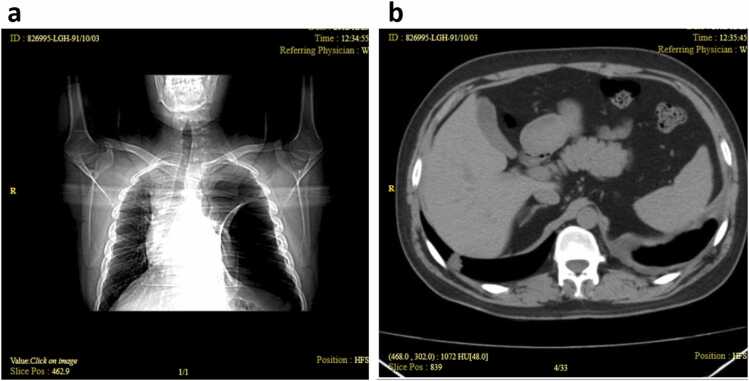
(A) Non-contrast axial chest CT scan at the level of the main bronchi showing a large left-sided pneumothorax, complete collapse of the left lung, and mediastinal shift to the right. (B) Non-contrast axial chest CT scan at the level of the left lower lobe demonstrating a thick-walled cavitary lesion containing an intracavitary soft-tissue mass (fungus ball). The surrounding crescentic rim of air (air-crescent sign) is characteristic of pulmonary aspergilloma.
